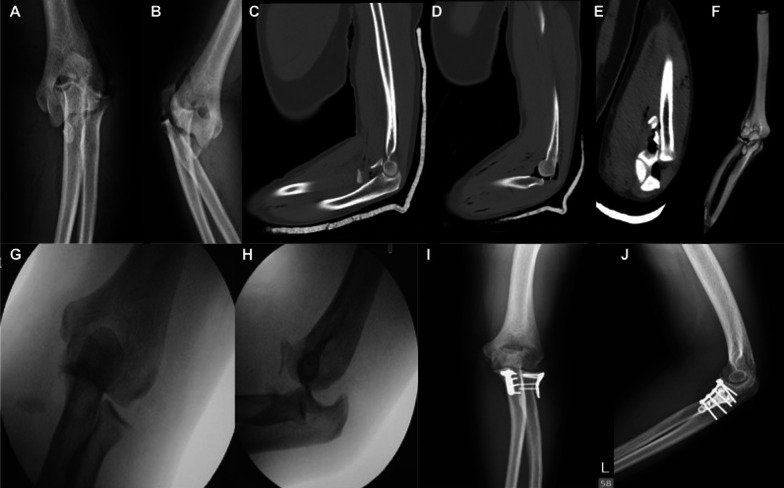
Case demonstration from our cohort. A, B Preoperative radiograph disclosed a 51-year-old patient with left terrible triad injury of the elbow; C–F computed tomography and three-dimensional reconstruction images revealed a Mason type II radial head fracture. The coronoid process fragment included the tip and anteromedial facet with less than 50% of the coronoid height (Regan–Morrey II) involved; G, H posterior subluxation of the ulnohumeral joint was observed during examination under anesthesia. I, J Radiograph at 2 years postoperatively. The patient first underwent coronoid process repair through an anterior approach. The radial head and lateral ulnar collateral ligament were addressed by the Kocher approach after coronoid process fixation
